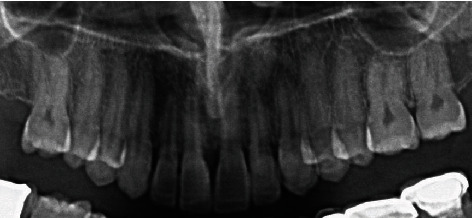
Radiographic photograph of veneers at a 12-month follow-up visit.
